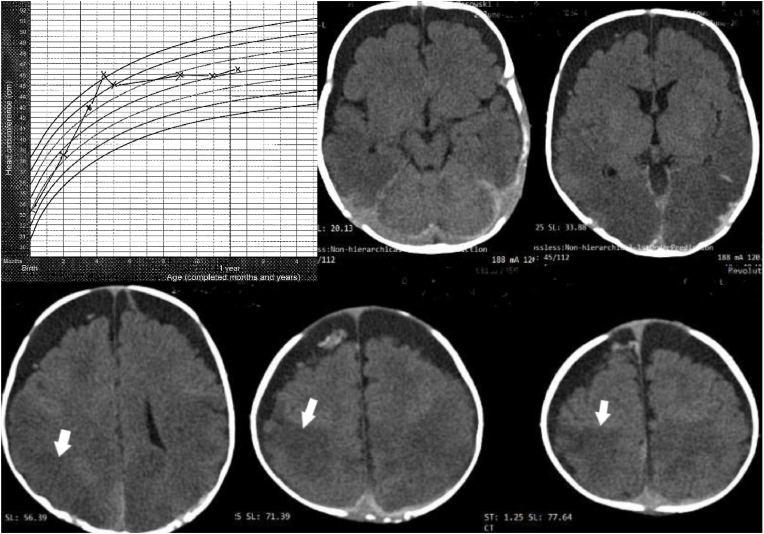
Case #2. Upper left: head circumference (HC) development from birth plotted on the WHO HC chart for boys shows a steep and too rapid initial HC increase – crossing several percentiles during the first four months, indicating hydrocephalus development [23]. Thereafter, there is a dramatic flattening of the growth curve – with no further growth from the 9th till the 11th month. This could be viewed as a normalization, but it is also possible – or even probable - that it is due to loss of brain tissue, see the final MRI scan in Fig. 3. In addition to bilateral subdural fluid collection with characteristics compatible with a chronic SDH and some minor components compatible with acute rebleedings within the right frontal chronic subdural hematoma (CSDH), these CT scans also show darker areas posteriorly in the brain parenchyma that may represent imminent brain damage (white arrows) – see also Fig. 3.
